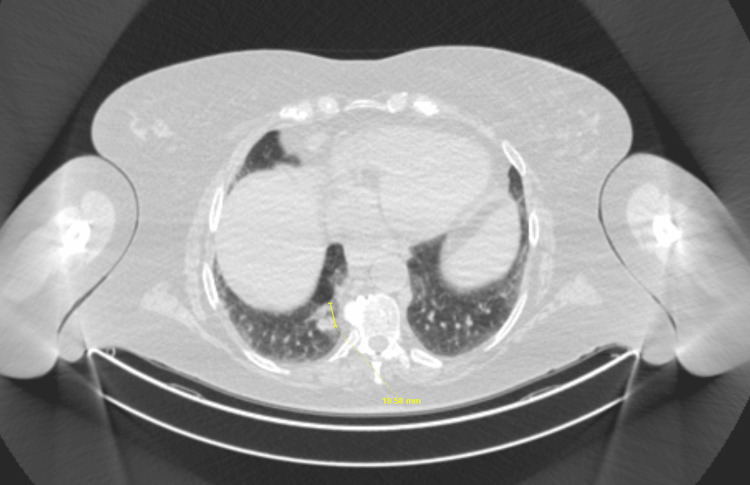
Corresponding CT scan with a yellow arrow showing incidental nodular lesions in the right lower lobe of the lung CT: computed tomography
